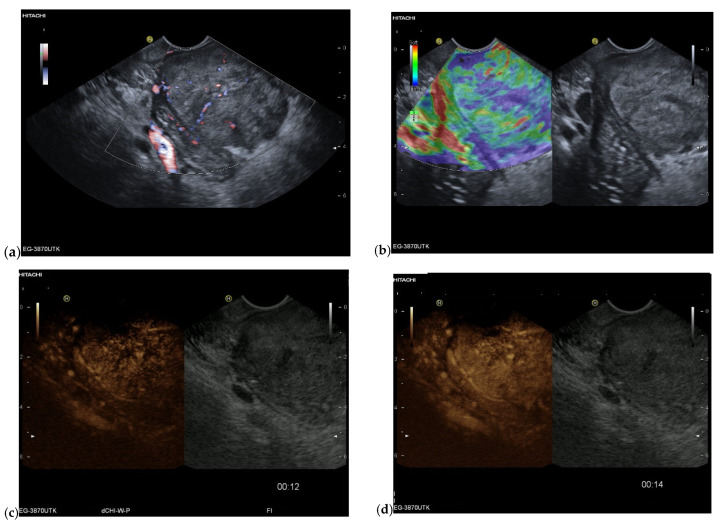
Metastasis of an endometroid adenocarcinoma in a female, 68 years old. Incidental finding of a mass at the pancreatic head, 1 year before an endometroid uterine carcinoma had been diagnosed and surgically operated. Endosonographically well vascularized lesion at the pancreatic head. Power Doppler imaging (a). In strain elastography of EUS, the lesion was predominantly stiffer, but also with softer portions (b). In CH -EUS with 4.8 mL SonoVue, the lesion was well vascularized in the arterial phase: first with an increased peripheral rim-like enhancement at 12 s and central nonenhancement (c), then heterogeneously hyperenhanced with emphasis of the periphery and small area of central nonenhancement at 14 s (d).
